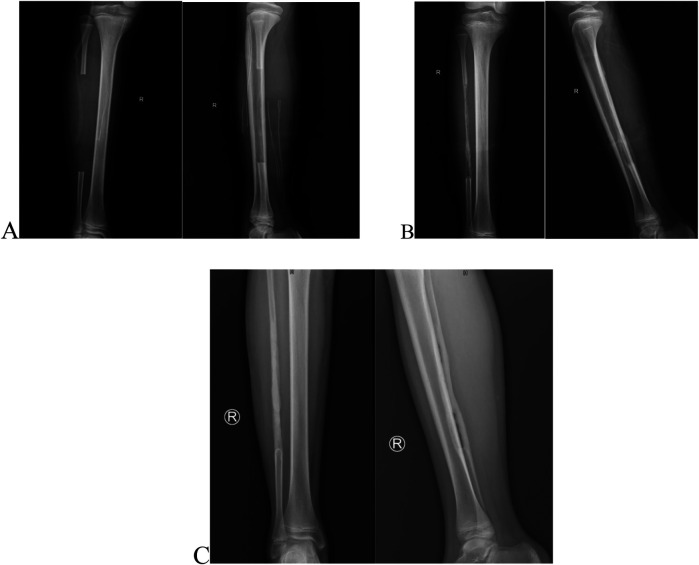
(A) Postoperative x-ray of the right tibia within 24 h after surgery: segmental fibular resection with periosteal preservation. (B) Two-month postoperative x-ray of the right tibia: Partial osteogenesis at the right fibula. (C) Nine-month postoperative x-ray of the right tibia: Extensive osteogenesis was observed in the right fibula, yet the medullary cavity was not fully formed.
